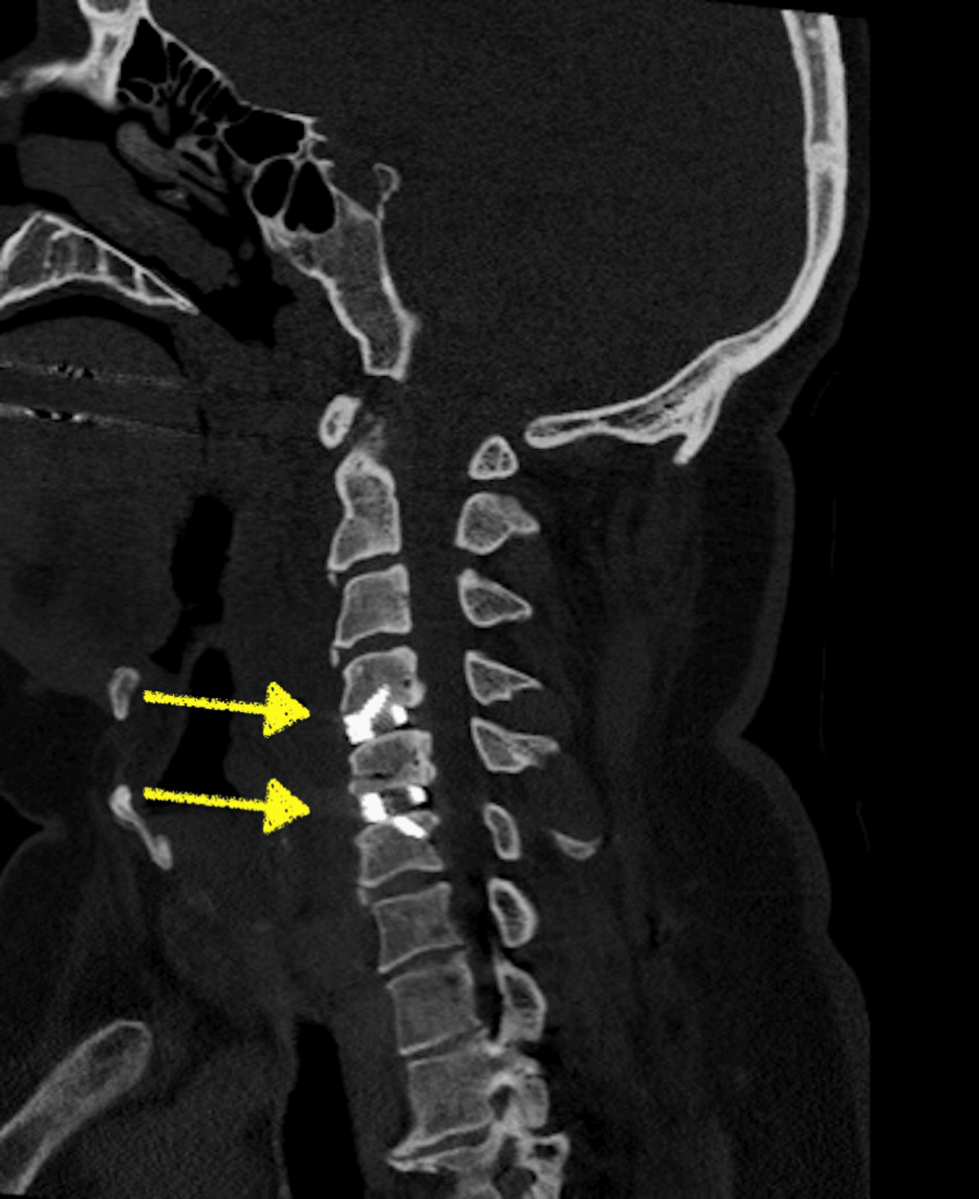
Sagittal view of non-contrast enhanced CT scan of the cervical spine further demonstrating proper screw placement (yellow arrows) at C4-C6 levels and absence of a hematoma formation.
